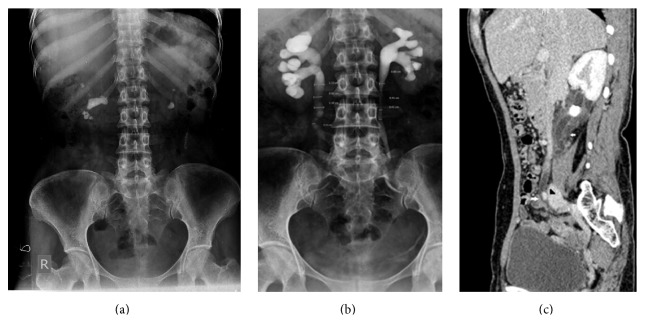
(a) Scout film: bilateral renal calculi. (b) IVU: a dilated right ureter, but the cause was not apparent. No ureteral calculus on noncontrast CT. (c) Contrast-enhanced CT scan: marked wall thickening in the region of ureteral dilatation (arrow) indicating ureteritis and also an extrinsic vascular compression of the right ureter (between arrowhead and lower white arrow).
